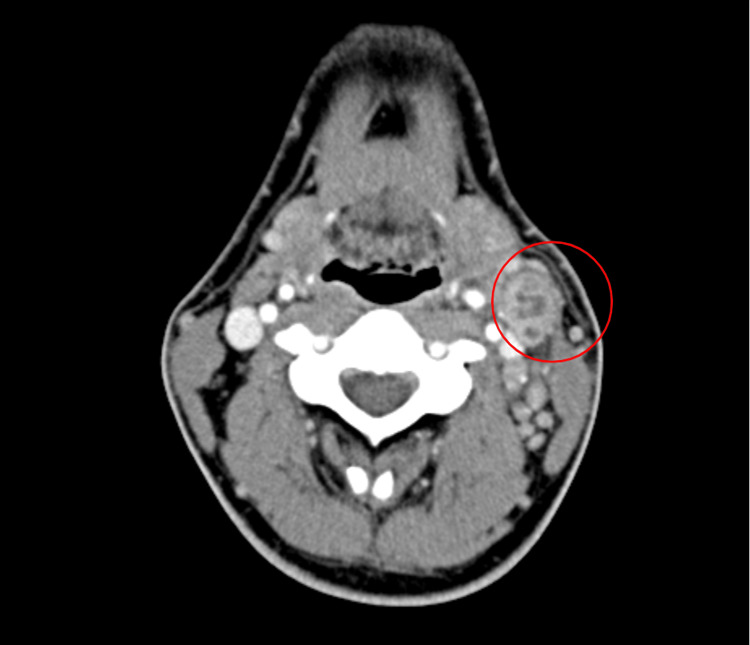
Initial neck axial view computed tomography scan with and without contrast The red circle demonstrates the mass of interest
